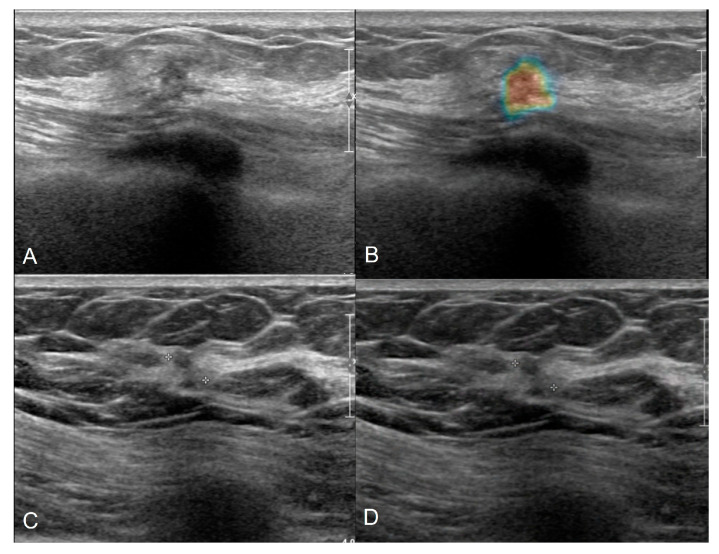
Ultrasound B-mode images show a lesion in a 65-year-old woman with mammographic density category C (A) with an AI true-positive assessment predicting a 62% probability of malignancy (C4C) (B), and a lesion in a 77-year-old woman with mammographic density category B (C) with a false-negative assessment predicting a probability of malignancy < 1% (C2) (D).
